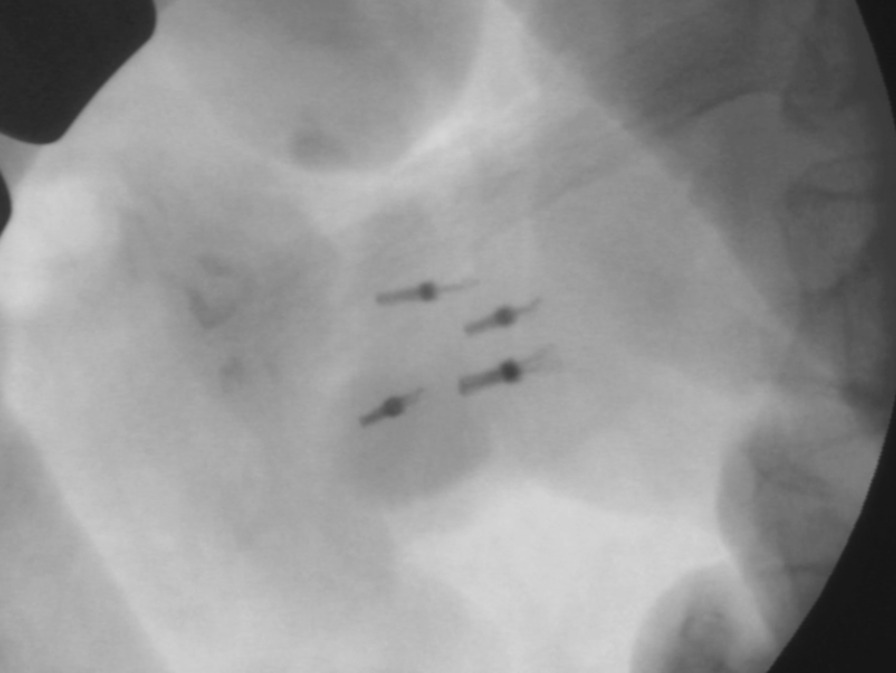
The clips on the margin of the tumor were visible under intraoperative X-ray imaging
